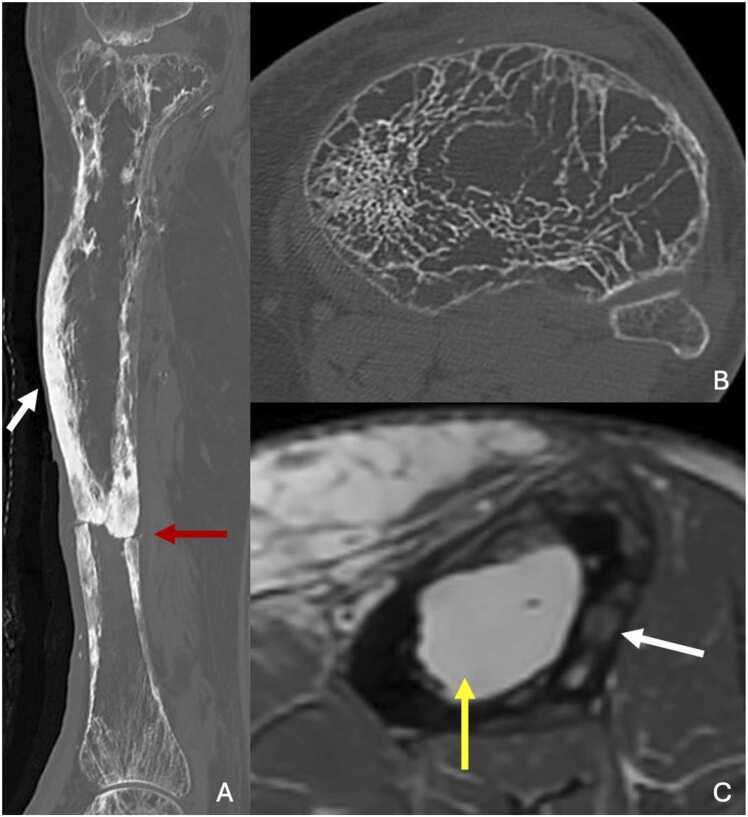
A. Paget’s disease of the right tibia in a 85-year-old-man. B,C. Paget’s disease of the left tibia in a 74-year-old-man (different patient). A. CT image in the sagittal plane showing a pathological fracture (red arrow) in a « saber-shaped » deformity of the tibia. Note the thickening of the anterior cortex (white arrow). B. CT image in the axial plane showing rarefaction and thickening of trabeculations of the epiphysis of the tibia. C. T1-weighted MR image in the axial plane showing a pronounced fatty signal of the medullar cavity (yellow arrow), cortical areas of resorption in hyposignal (white arrow).
